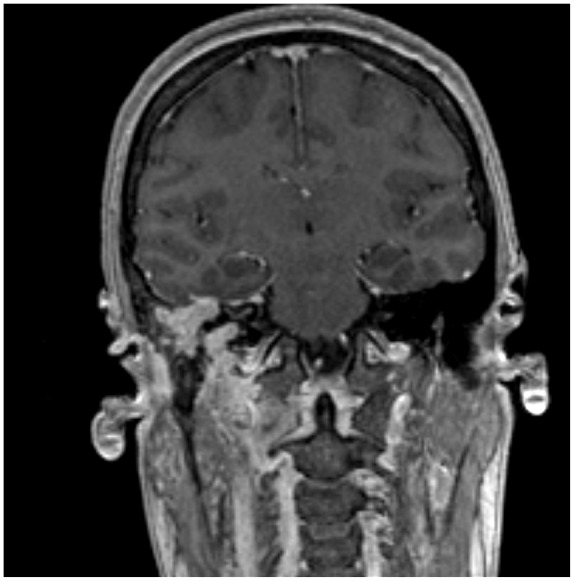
Coronal post-contrast magnetic resonance imaging shows homogenously enhancing soft tissue mass in the right mastoid air cells, extending along the tegmen tympani, associated with reactive dural thickening.
